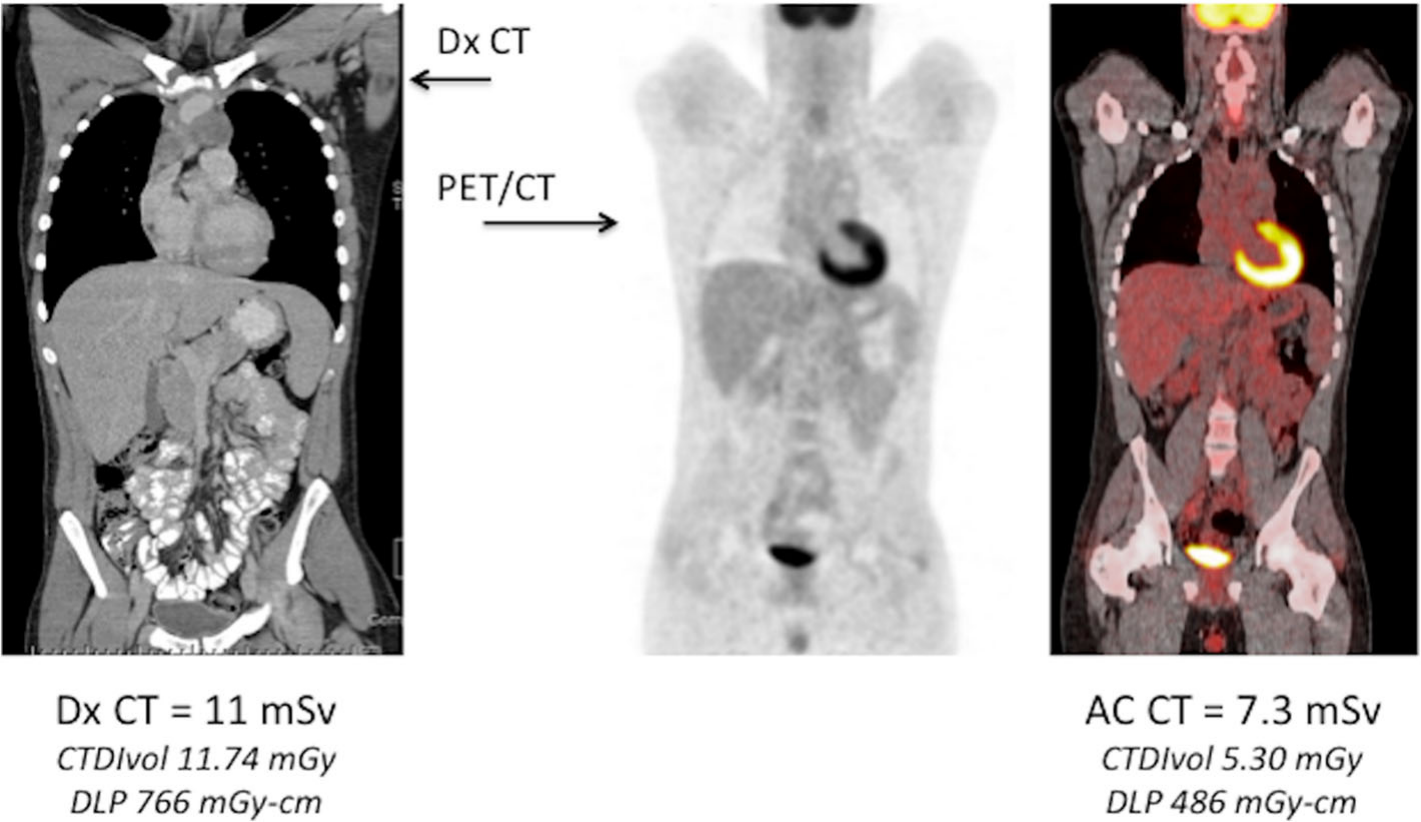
The Double Torso Exam: PET/CT + Diagnostic CT. Dx CT and PET/CT exams performed on the same patient with Hodgkin lymphoma. Both examinations were performed during the same appointment, following 2 cycles of chemotherapy, and show similar coverage between the two CT exams. In this example, the AC CT dose is approximately 2/3 of the Dx CT dose. Eliminating one of the two CT exams and performing a single CT, offers an opportunity for significant CT dose reduction
